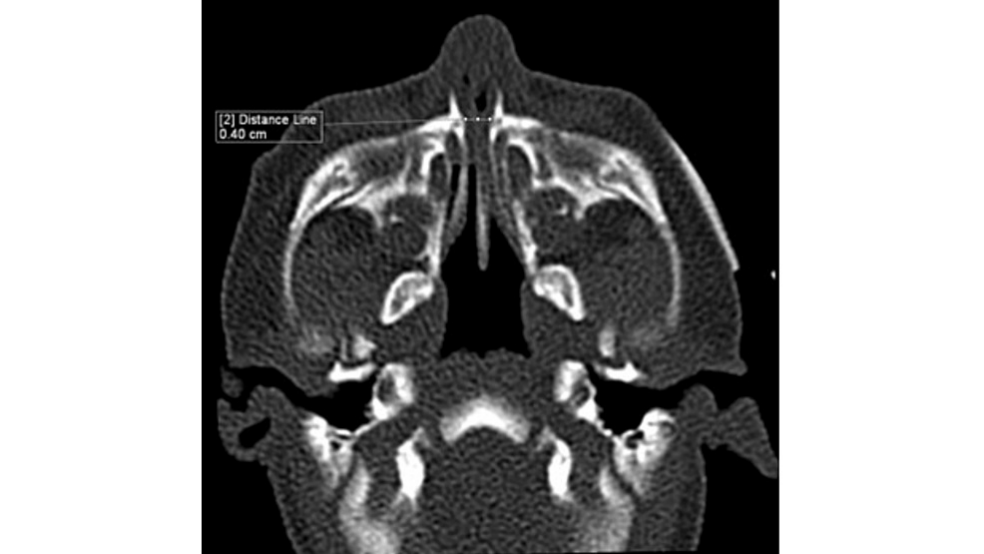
Axial CT scan shows the width of the pyriform aperture (4 mm).
